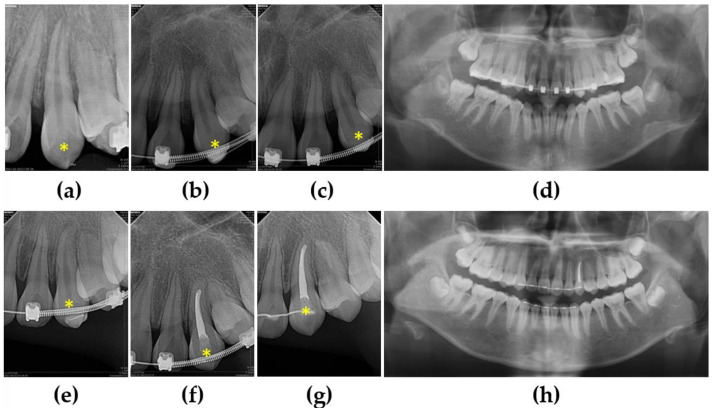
Radiographic examinations. The transplanted tooth is indicated with a yellow asterisk (*). Radiographs obtained on the day of autotransplantation (a), 1 week (b), 1 month (c), 3 months (d,e), 5 months (f), 14 months (g), and 2 years (h) postoperatively.
